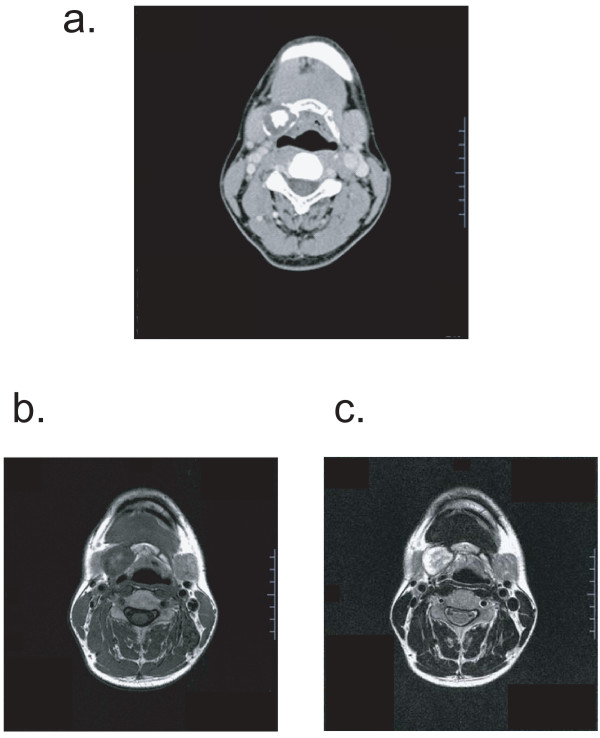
Preoperative imaging by CT and MRI. (a) Computed tomography of his neck. A gourd-shaped distension of bone was present on the right side of his hyoid bone. A multilocular cystic area of low density was observed in the distention. Central ossification was evident in the anterior lesion. (b) Magnetic resonance imaging T1-weighted image with low signal intensity. (c) MRI T2-weighted image high signal intensity. A mosaic pattern was visible in the high signal area.
